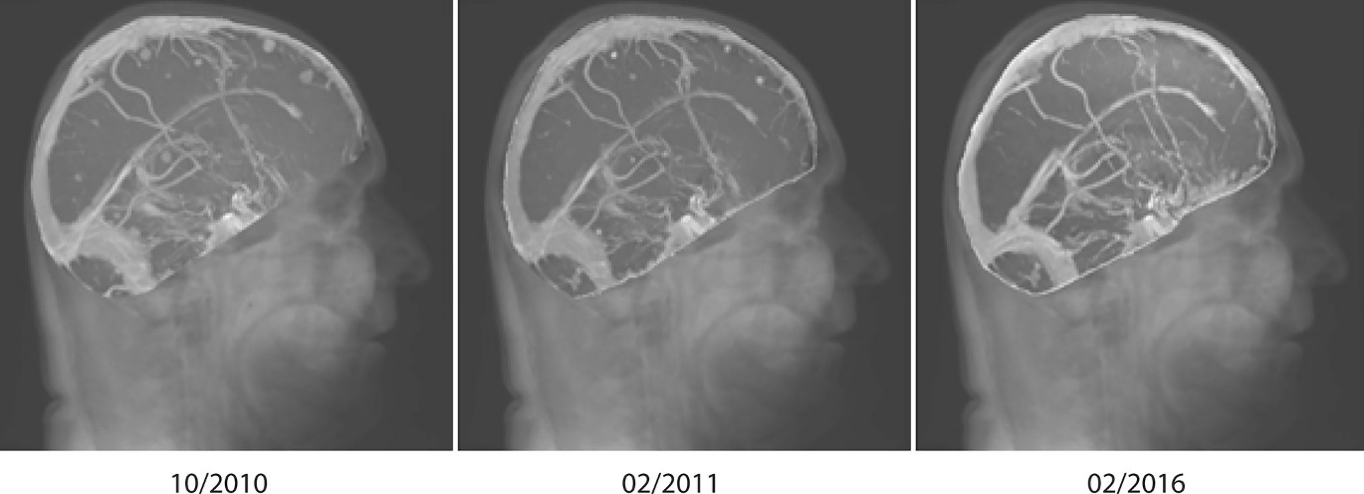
Maximum intensity projection (MIP) view of T1-weighted magnetic resonance images (MRI) immediately following whole brain irradiation (WBRT; 10/2010), 1 month after completion of 4 cycles of ipilimumab (IPI; 02/2011) and 5 years later (02/2016), showing complete remission (RECIST 1.1) of brain lesions
